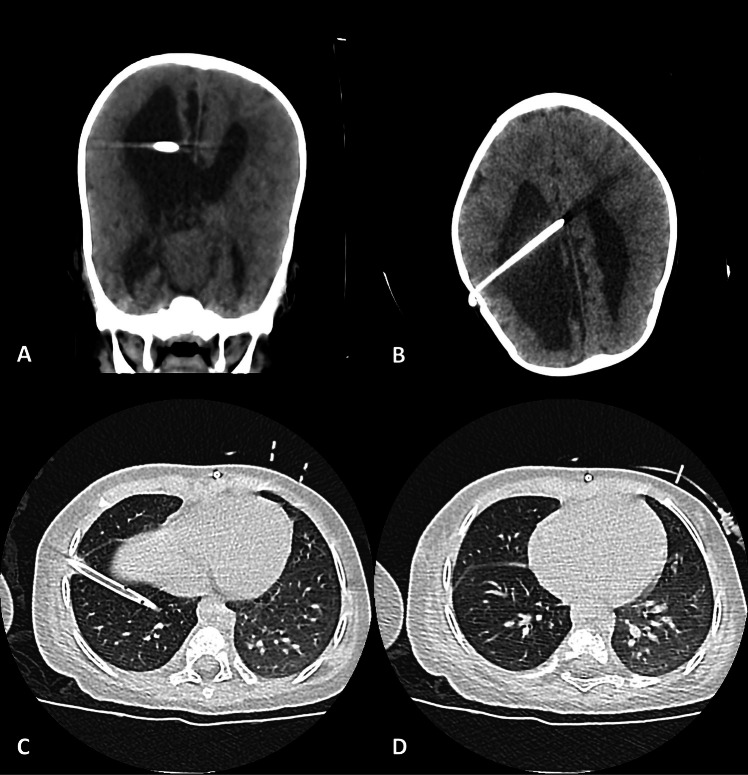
Postoperative CT scan, after the catheter was reinserted over the sternum. A Coronal reconstruction and (B) axial view of the cranium, demonstrating the placement of the ventricular catheter inside the right ventricle. C and D Axial chest CT scan showing no remaining pleural effusion, with the thoracostomy drain still in place (visible in C)
